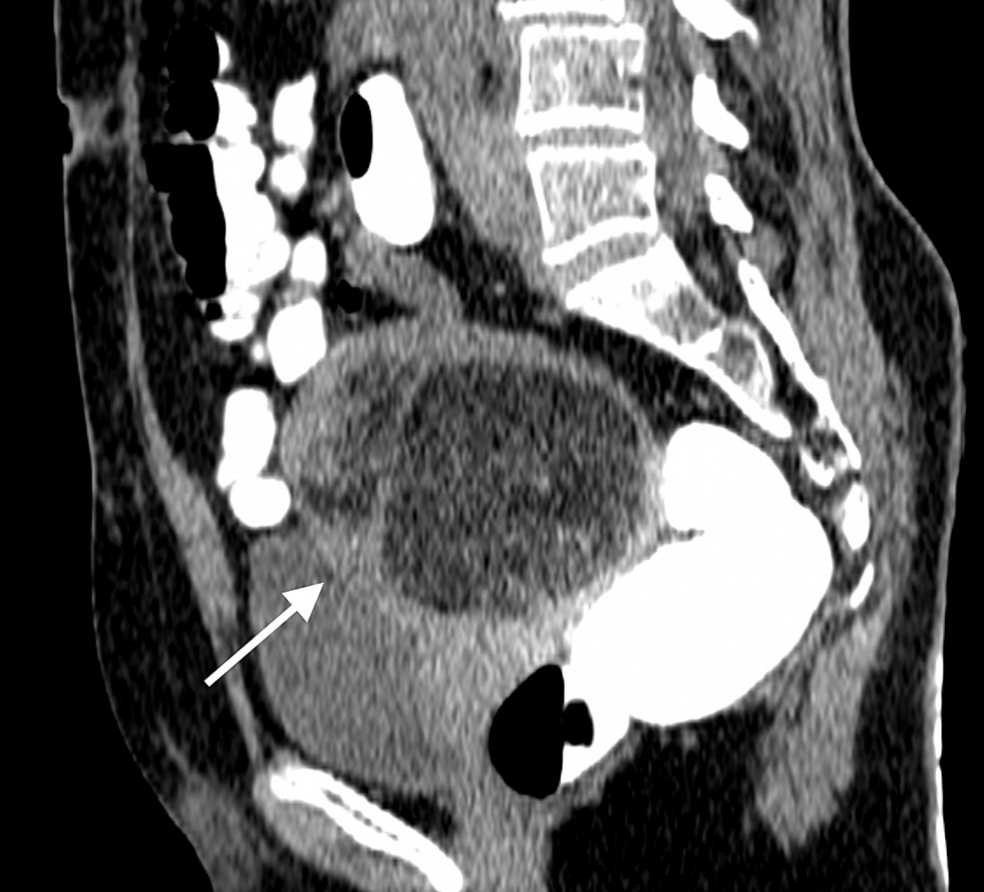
Sagittal CT reformat image with rectal contrast, showing the fat and soft tissue density uterine mass (white arrow) located anterior to the rectum and indenting the posterior wall of the urinary bladder.
